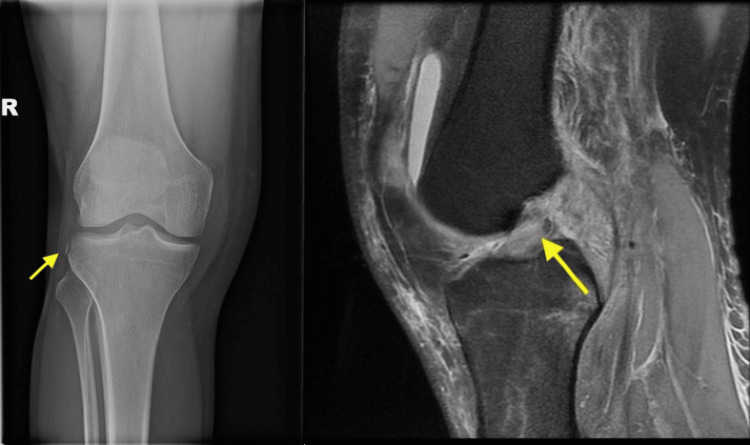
Anteroposterior (AP) radiograph of the lateral tibial plateau (left, panel A), MRI of the knee (right, panel B). A plain anterior-posterior radiograph showed an avulsion fracture of the lateral tibial plateau (Panel A). An MRI, completed as an outpatient, confirmed a full-thickness ACL rupture, and a concurrent MCL rupture was noted on the MRI (Panel B).
